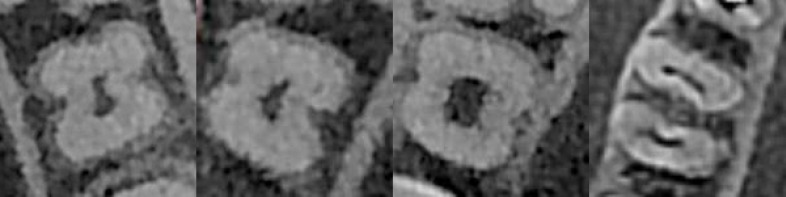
Examples of transverse CBCT sections
